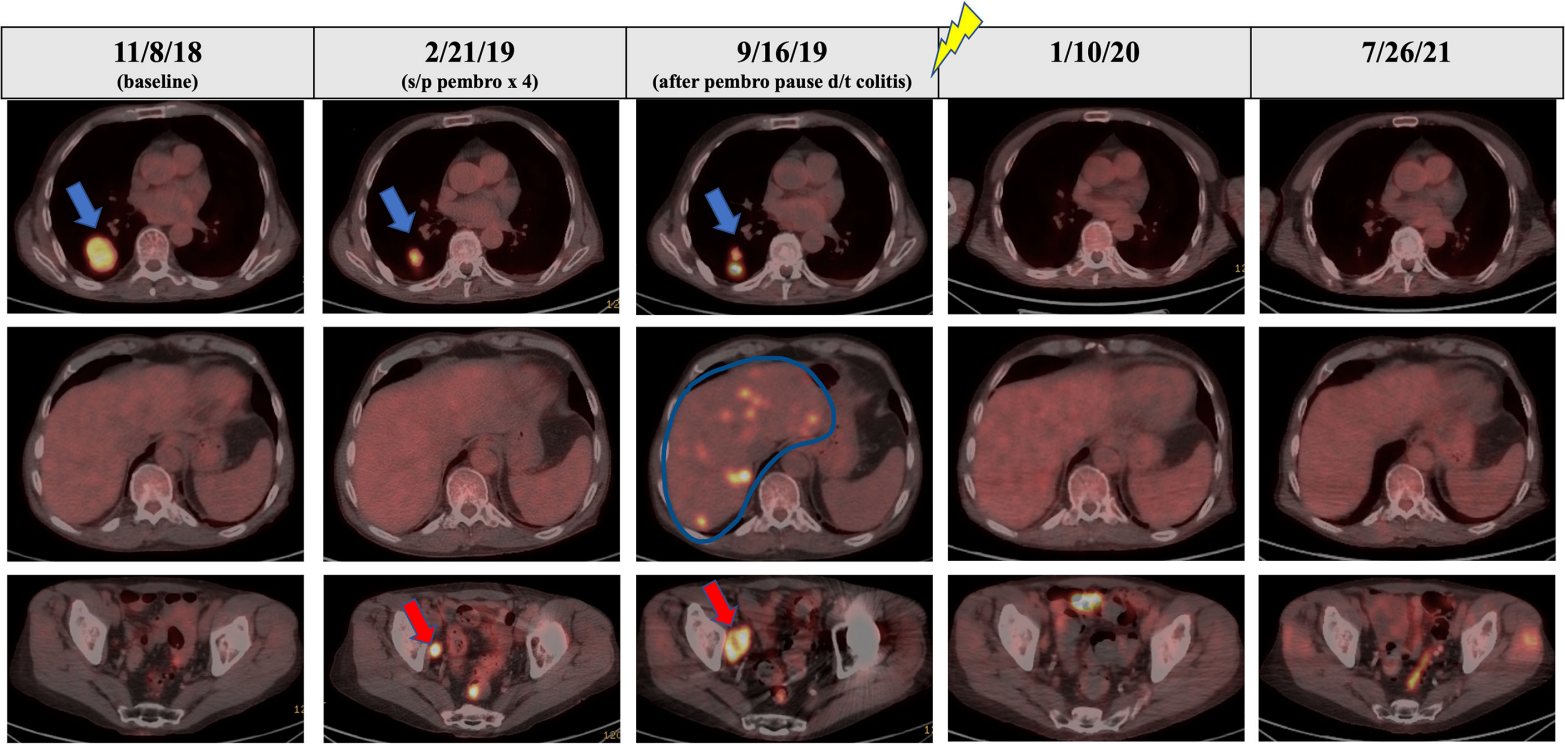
PET/CT scans over the course of the patient’s treatment. The blue arrow in the top panel shows RLL nodule with good response to initial pembrolizumab monotherapy from the baseline scan in November 2018. The red arrow in the bottom panel shows a site of progression in the right pelvic sidewall through initial pembrolizumab monotherapy representing mixed response to initial pembrolizumab monotherapy. Palliative RT was delivered October 2019 to sacral metastasis (signified by lightning symbol). The blue line in the middle panel outlines multifocal progressive disease in the liver seen on the September 2019 PET/CT after break in therapy due to pembrolizumab-related colitis. PET/CT in January 2020 and September 2021 show complete metabolic response.
